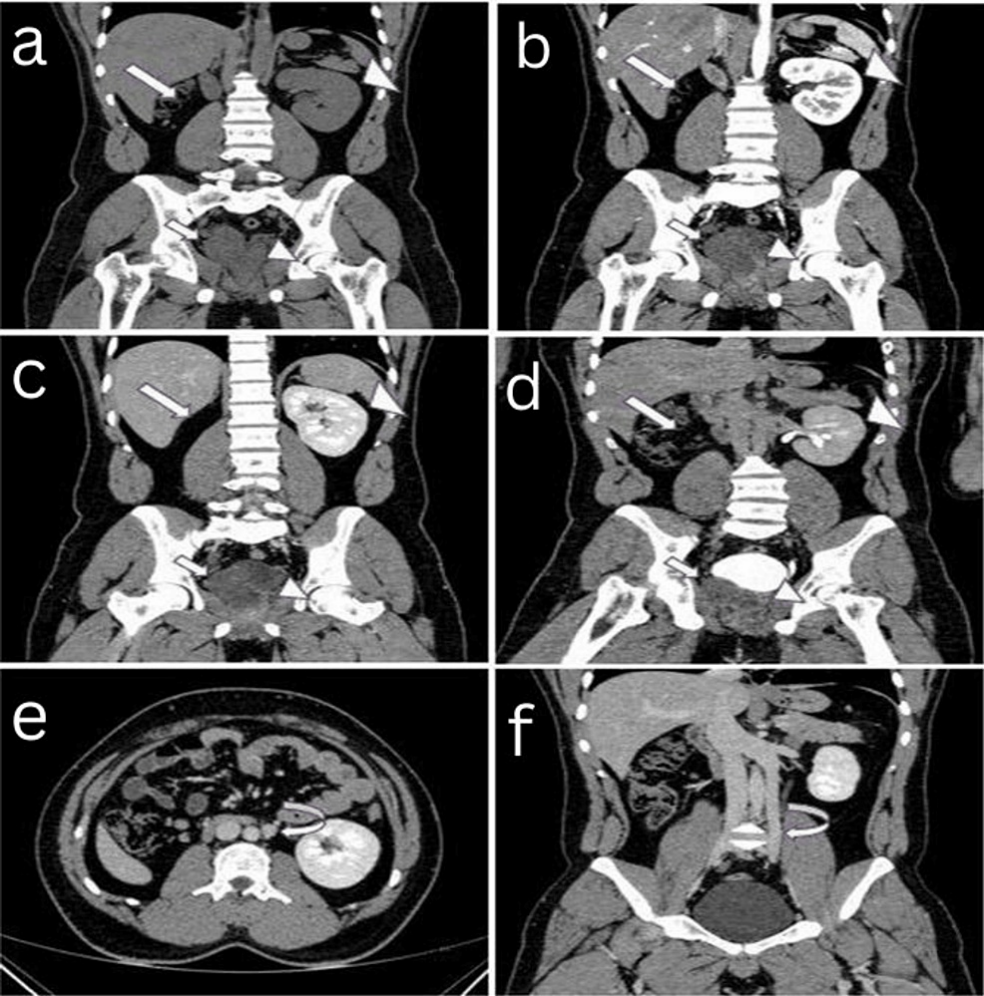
A 20-year-old male presented with a history of recurrent lower urinary tract infections associated with prostatism, urinary urgency, frequency, dysuria, pelvic/perineal discomfort, and scrotal pain over the past three months. (a) Plain CT of the abdomen in coronal view; (b-d) CECT abdomen in coronal view (cortico-medullary, nephrographic, and excretory phases) showed non-visualization (absence/agenesis) of the right kidney in the right lumbar region (large thick white arrow), right seminal vesicle cyst (small thick white arrow), left kidney was normal (large white arrowhead), left seminal vesicle was normal (small white arrowhead); (e,f) CECT abdomen in axial and coronal views (nephrographic phase) showed duplicated inferior vena cava (curved white arrow). CT: computed tomography CECT: contrast-enhanced computed tomography
